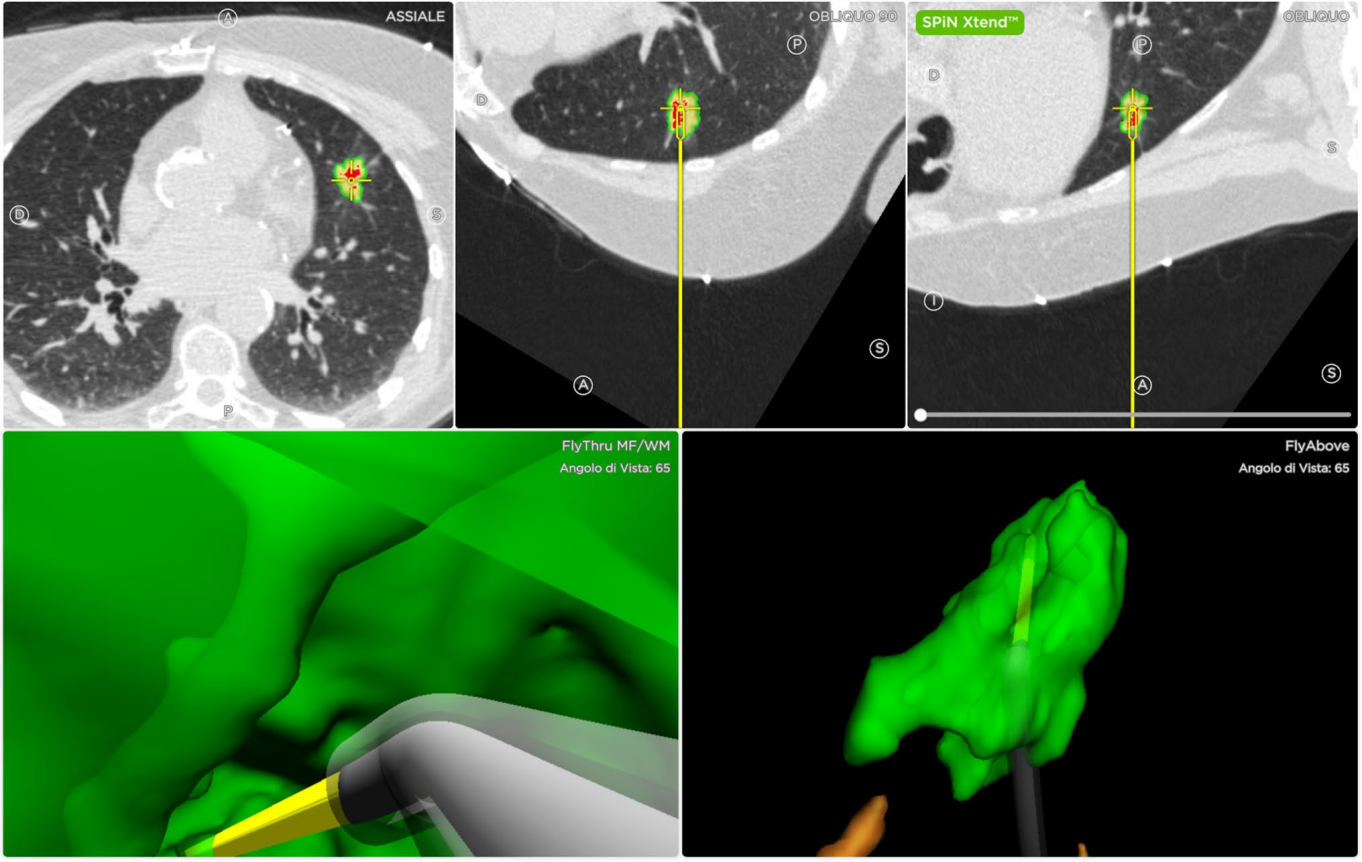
Multiplanar Computed Tomography images (upper images), 3D images of the real-time sampling with transbronchial needle aspiration (lower images)
